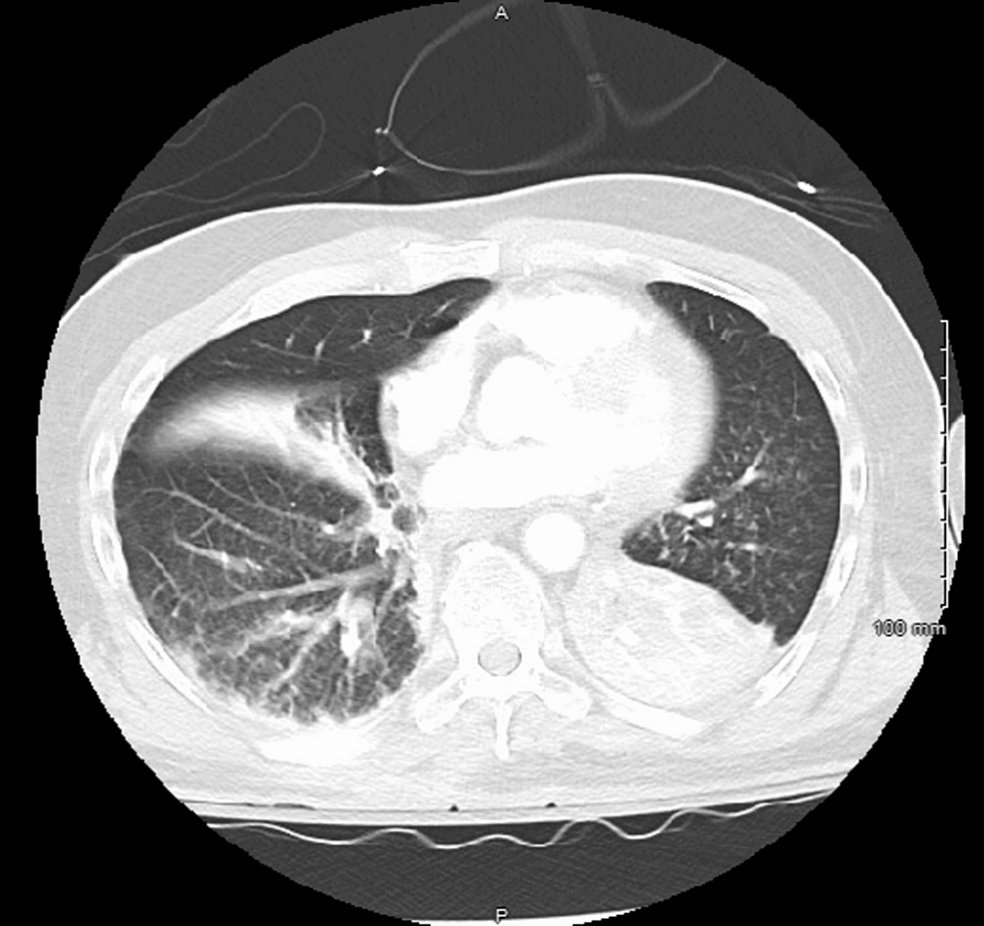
CT chest pulmonary angiogram shows atelectasis in the right middle lobe and bilateral lower lobes, significantly worse in the left lower lobe
